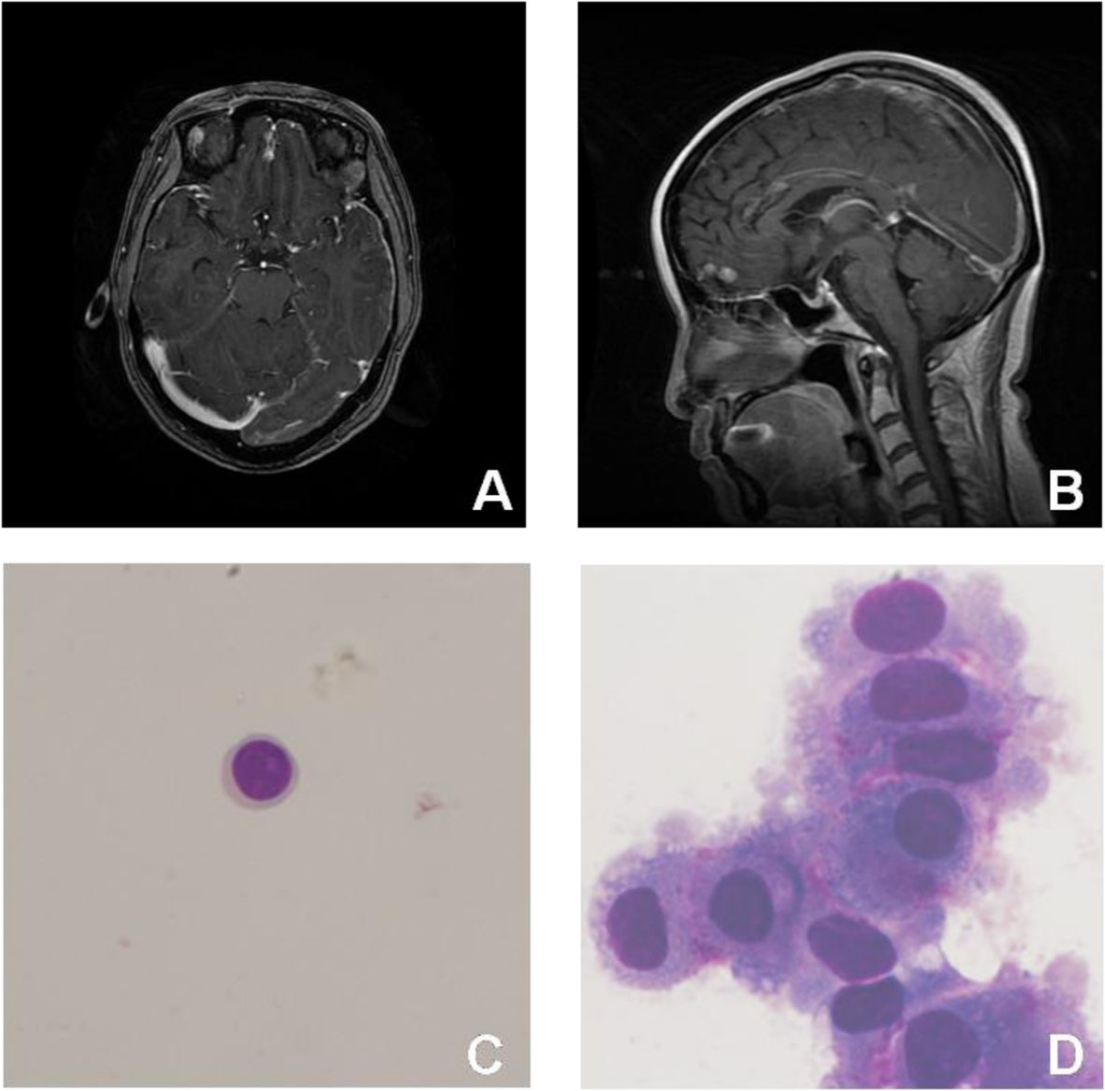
Head contrast enhanced MRI (a and b) of one patient showed abnormally uniform leptomeningeal enhancement. At the same time, no neoplastic cells were found in CSF by CSF cytology (c) while CSF sample revealed ctDNA and showed cancer-associated gene mutations of PTEN K13Q (0.464%), TP53 P301Q (0.809%), VHL R161Q (0.213%), CDKN2A A68T(0.235%) and FGFR2 Y375C (0.207%) by NGS. CSF cytology (d) 1 year later showed a large number of neoplastic cells
